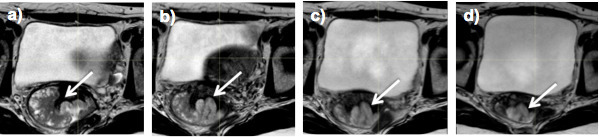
Changes in cervix tumour volume (arrow), as seen on weekly MRI during treatment at (a) week 0, (b) week 2, (c) week 3 and (d) week 4.
